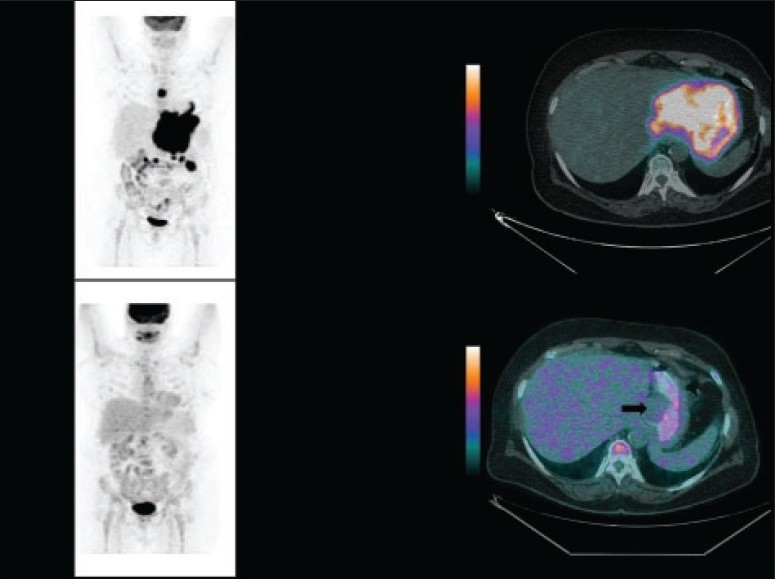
A 66-year-old woman diagnosed with Hodgkin lymphoma. The 18F-FDG PET/CT study (left and right upper panels) for initial staging showed nodal involvement above and below the diaphragm. 18F-FDG PET/CT after four cycles of chemotherapy (left and right lower panels) showed complete metabolic resolution of the disease with small non–FDG-avid residual soft tissue (black arrow on the fused image).
